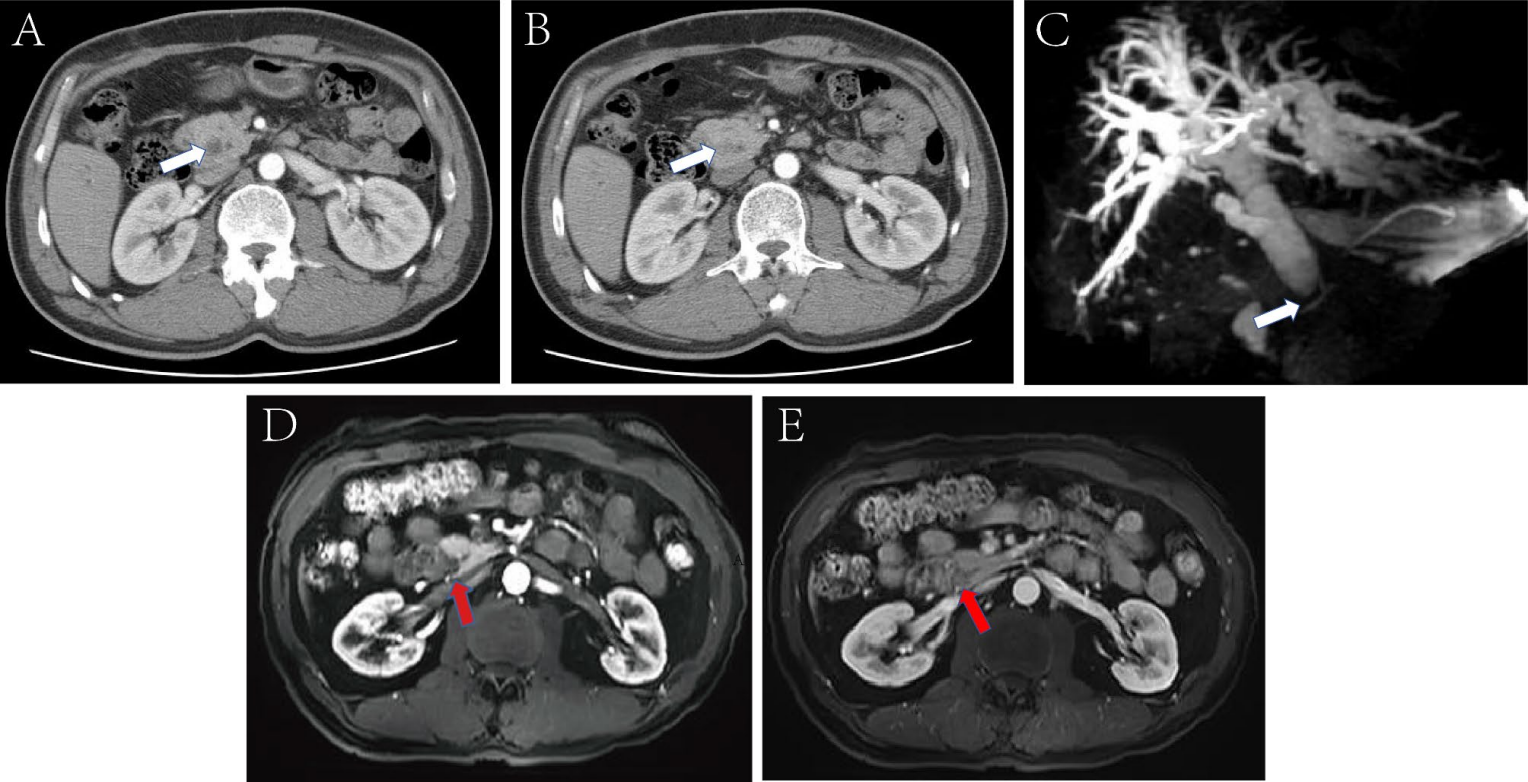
(A, B, C) are CT and MRCP images of a 52-year-old patient with AH of the distal bile duct with cholangiocarcinoma: showing marked dilatation of the bile duct and truncation of the distal bile duct (white arrow), with no obvious occupying lesions. (D, E) are MRI images of a 66-year-old patient with AH of the distal bile duct: An approximately 16*17 mm abnormal oval signal shadow(red arrow) is seen in the ampulla of Vater, part of the lesion is poorly demarcated from the head of the pancreas, and dynamic enhancement scans show mild enhancement. (C) Arterial phase; (D) Balanced phase
